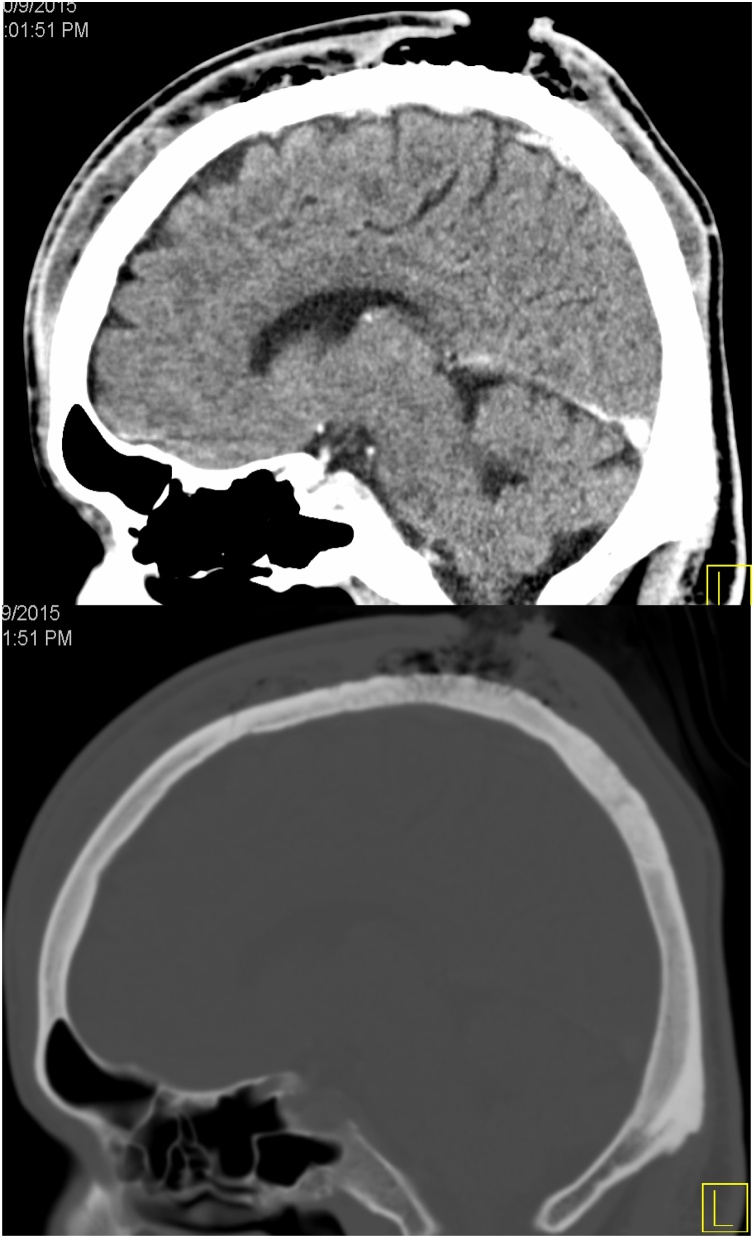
Head CT showing subgaleal collection, the bony wound shows involvement of cranial bones.
